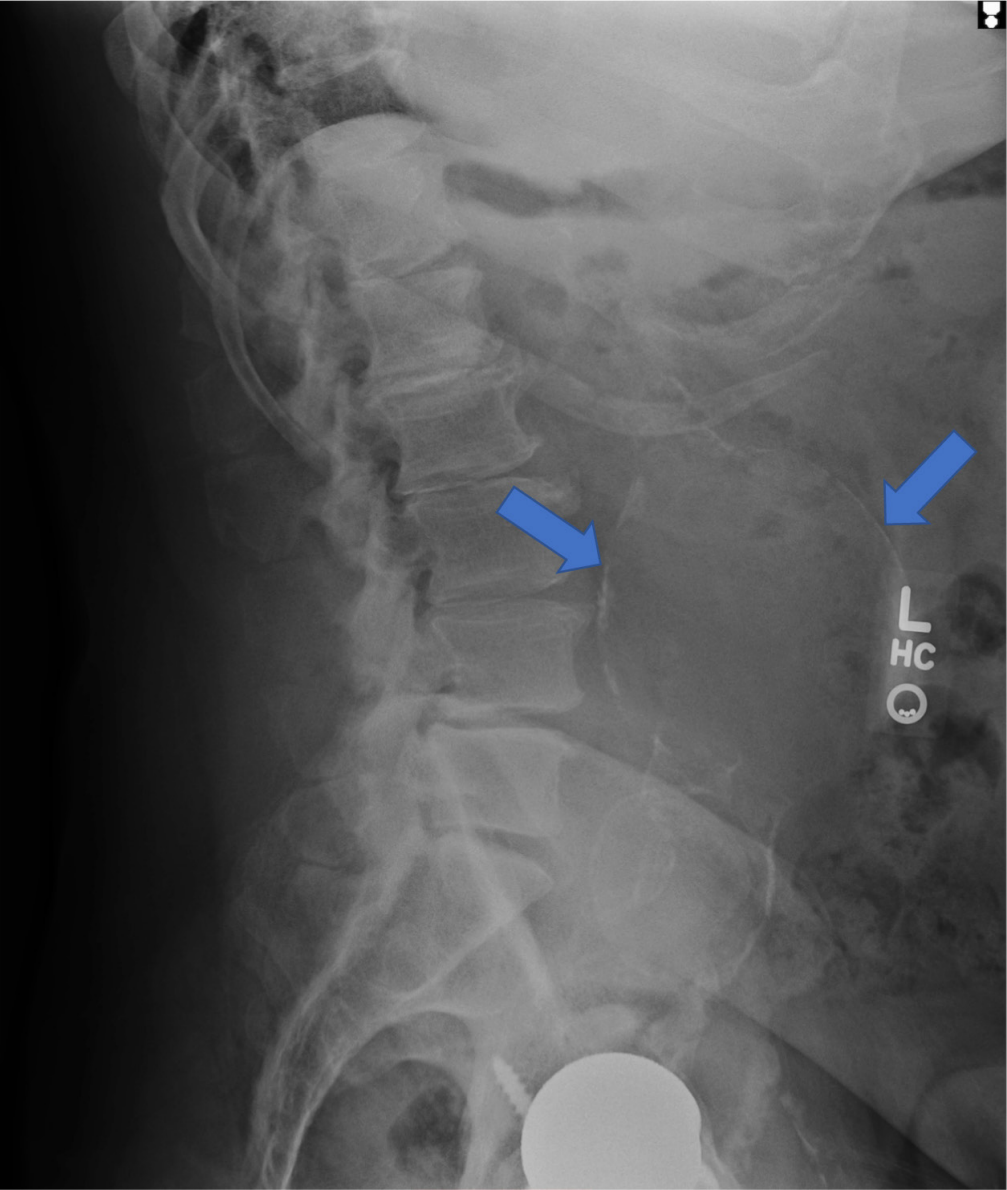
Lateral radiograph of lumbar spine demonstrating calcified outline of abdominal aortic aneurysms (blue arrows).
